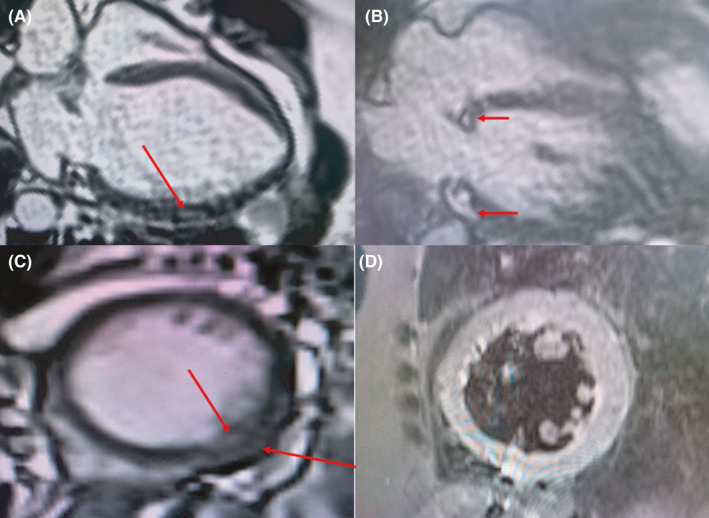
Cardiac MRI images showing patchy late gadolinium enhancement of the basal septum and mid to apical lateral LV wall A, basal septum and basal lateral wall B, and mid‐inferolateral wall C. The absence of edema or inflammation is shown in STIR images D, after treatment
